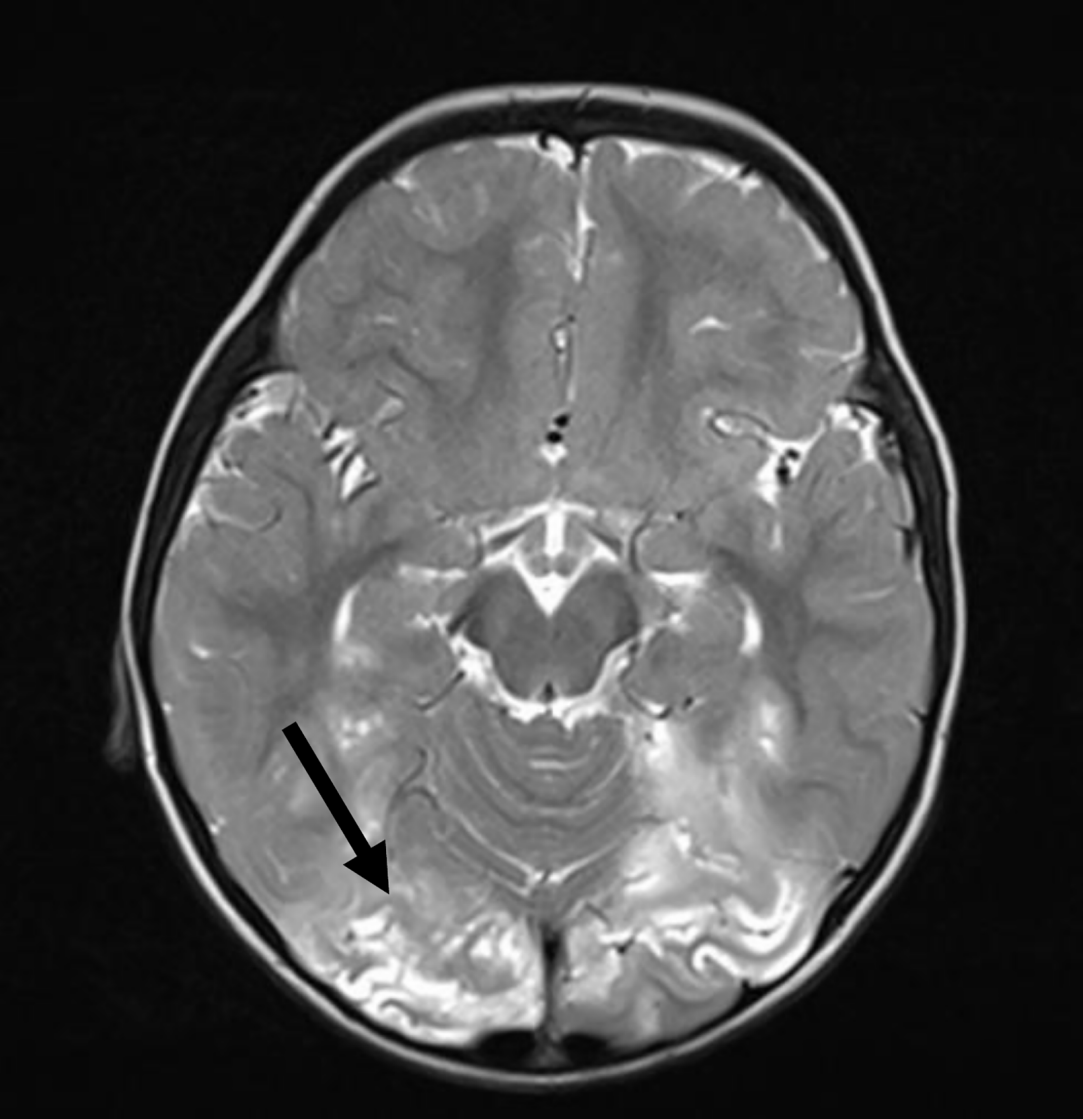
Magnetic resonance imaging shows T2-weighted cortical and subcortical hyperintensities in bilateral occipital region.
